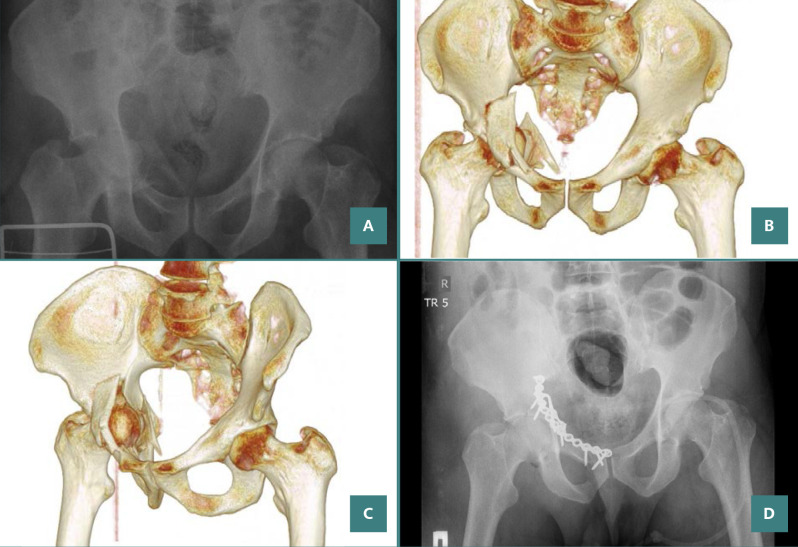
A 42-year-old patient with a both-column fracture of the acetabulum. Preoperative X-ray and CT sections: (A) preoperative direct X-ray, (B,C) preoperative CT sections, D) X-ray on postoperative day 1.
